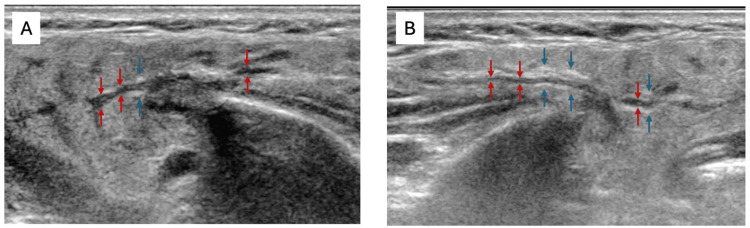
Ultrasonographic appearances of parotid salivary glands The right (A) and left (B) parotid glands show similar ultrasonographic appearances, with ductal dilatation extending throughout the gland (red arrows). The main dilated ducts are surrounded by thick hyperechoic tramlines, suggestive of fibrosis (blue arrows). The background gland parenchyma is almost normal.
